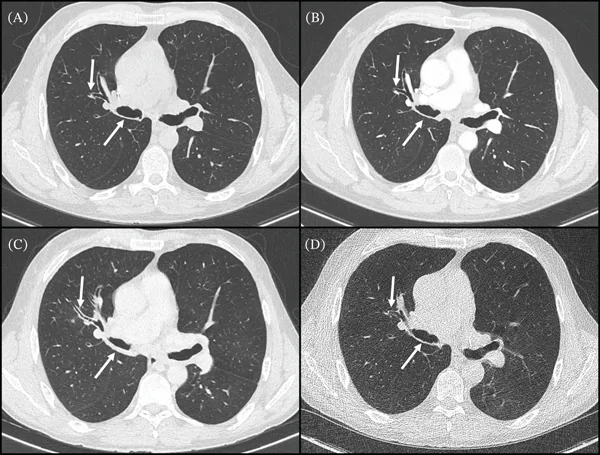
Axial and coronal CT imaging of the chest. (A) December 2018—7 months after transplant with normal bronchial wall diameter (see arrow). (B) July 2019—13 months after transplant with stable bronchial wall diameter. (C) February 2024—marked progression in bronchial wall thickening. (D) April 2025—improvement in bronchial wall thickening after 14 months of treatment with infliximab closer to pre‐treatment baseline.
